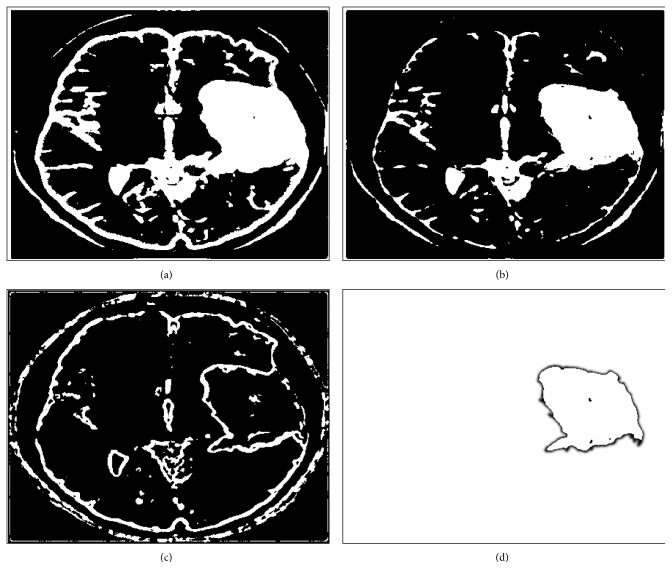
Example of steps in segmentation (tracing) by expert of the gray and white matter regions in brain tumor MRI images in a sample patient brain MRI image.
